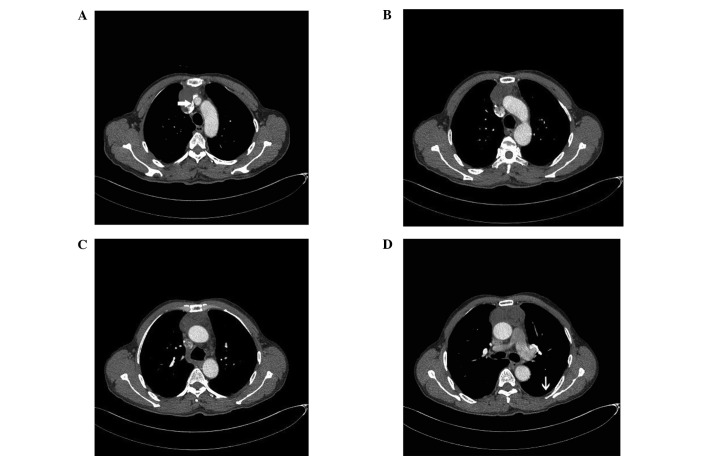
Enhanced computed tomography shows an anterior mediastinal mass (8.3×6×3.5 cm) with heterogeneous contrast enhancement, with an unclear plane separating it from (A) the constricted left innominate vein (white arrow) and (B and C) the aorta and the superior vena cava, and with (D) a trace of pleural effusion (white arrow).
